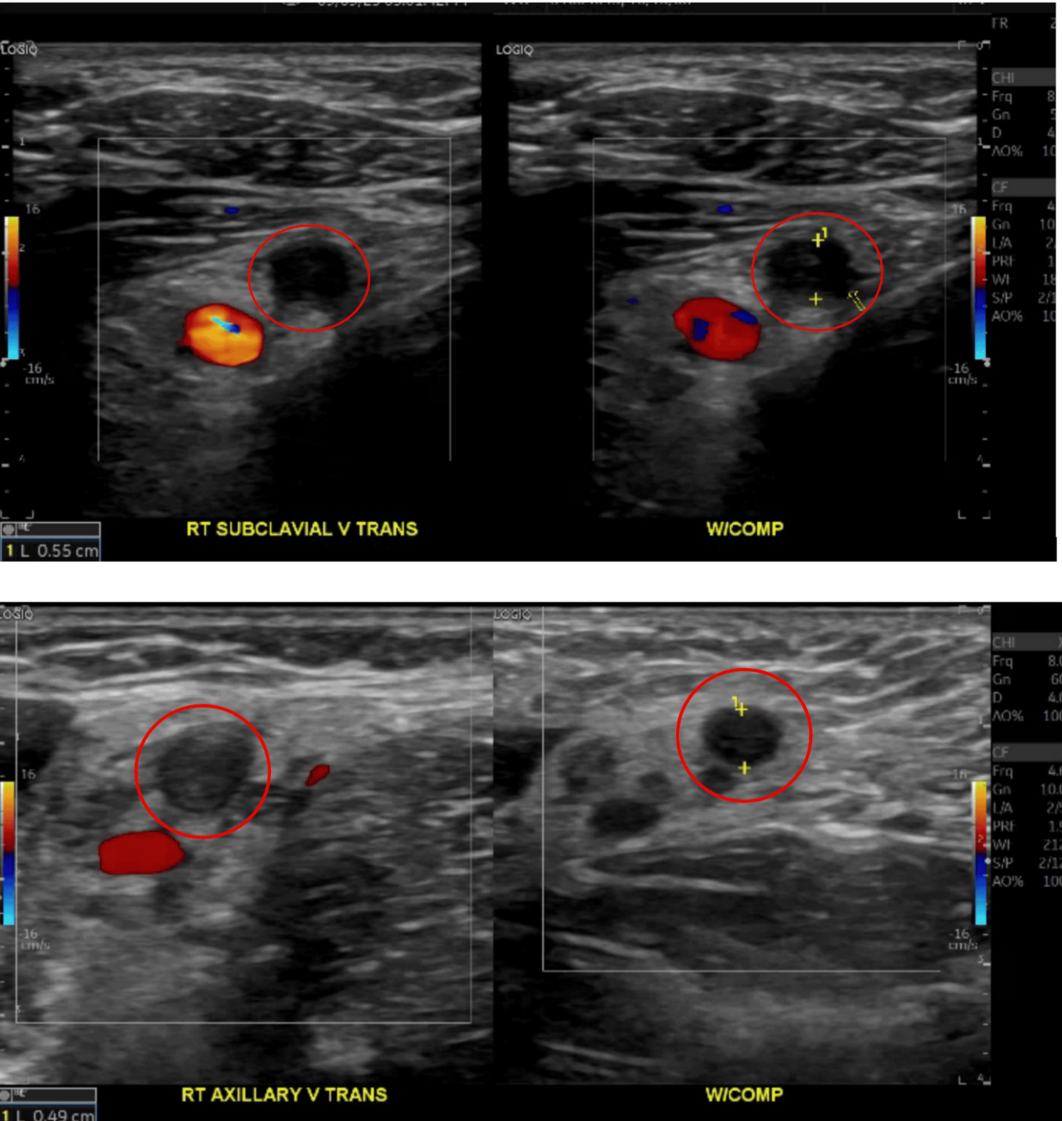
Duplex ultrasound revealing occlusive thrombosis of the right subclavian and axillary veins highlighted by the red circles with minimal compression shown on the right, signifying acute thrombosis
